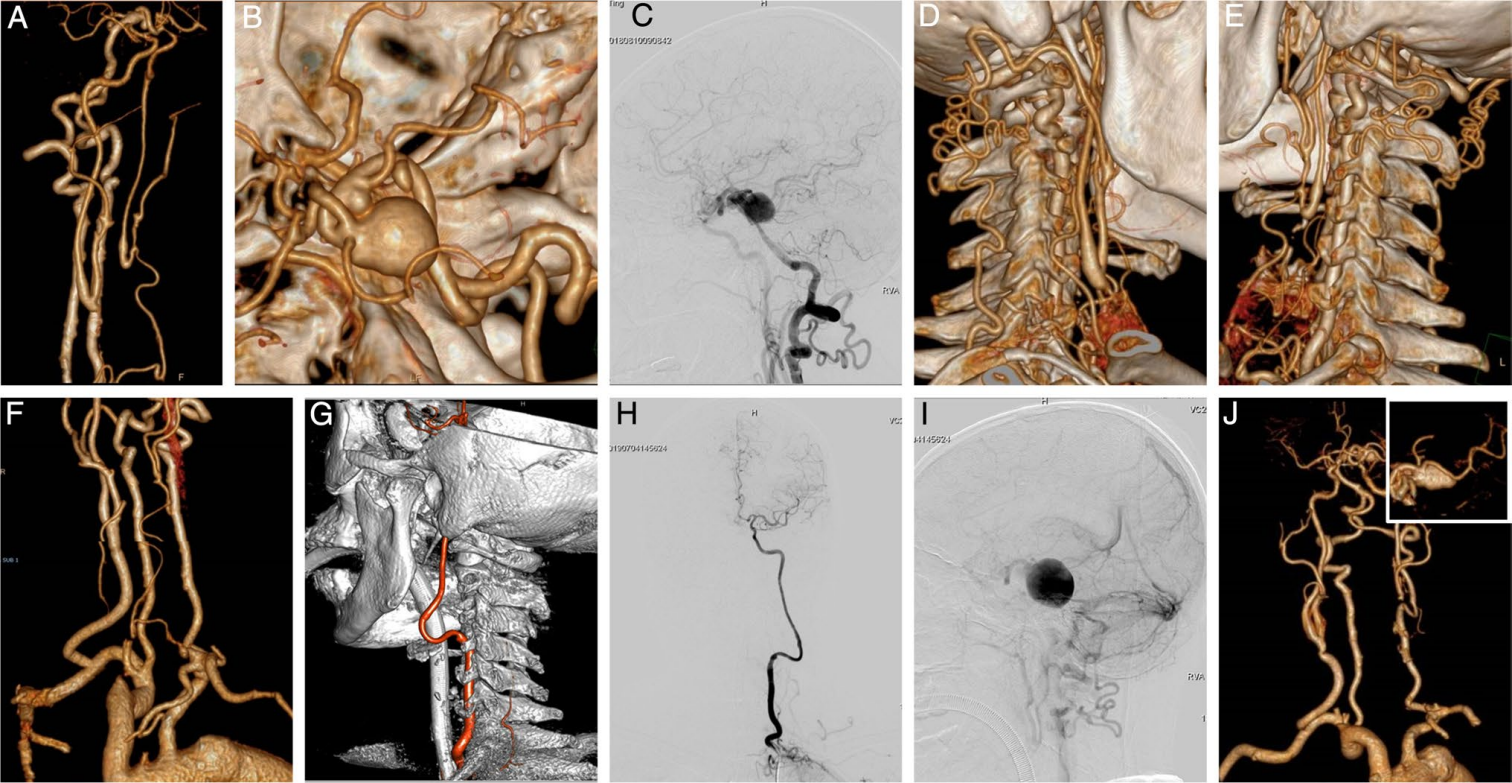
A case of compensatory terminal BA aneurysm (Type III). A–B: CTA confirms bilateral CCA occlusions and a giant terminal BA aneurysm involving the left PCA. C: Angiography shows flow diversion via the left PCOM and ACOM, contributing to aneurysm development. D–E: CTA reveals opened cervical collateral pathways via OA, AA/DA, and SBA branches. F: One-year patency of the venous graft bypass for right CCA reconstruction. G–H: Intraoperative angiography confirms graft patency with robust filling of the left ICA territory. I: Contrast retention is observed within the aneurysm sac. J: Follow-up CTA demonstrates progressive aneurysm shrinkage
